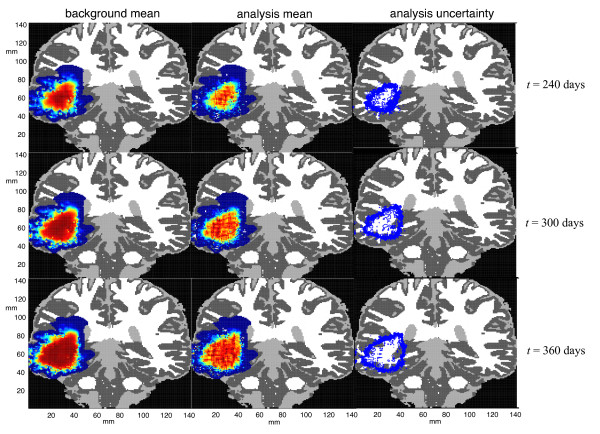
Example of forecast correction. The paneels show how the LETKF data assimilation algorithm corrects the background forecasts in Experiment 2 at *t *= 240, 300, 360 days. The left column shows the mean of the 60-day background forecast mean for the indicated period and the middle column shows the analysis mean after the synthetic noisy MR imaging data have been assimilated. The right column shows a "spaghetti plot" showing the contour, for each of the 50 ensemble members, where the total tumor cell density is one-half of its maximum value.
